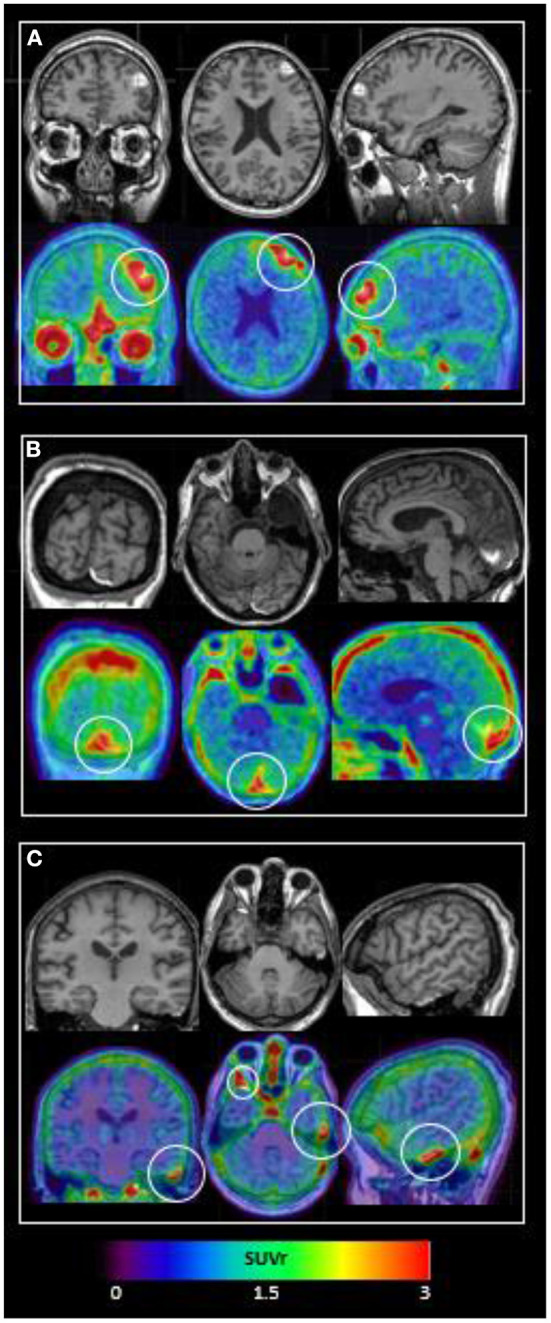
^18^F-MK6240 PET and MRI performed within 14 days of complicated mild TBI in 3 subjects. Top panel shows T1 MRI and bottom panel shows ^18^F-MK6240 PET (SUVr) overlaid on MRI. (A) Subject #1: MRI shows hemorrhagic contusions in L frontal and L>R orbitofrontal regions. PET shows focal radiotracer accumulation within and surrounding these regions, with the L frontal lesion (white circle) showing a c-shaped configuration. (B) Subject #2: MRI shows parafalcine trace subdural hematoma, diffuse atrophy, and a developmental arachnoid cyst. PET signal in the region of subdural hematoma (white circle) may be present, but is difficult to differentiate from (normal, off-target) signal in adjacent dura. (C) Subject #3: MRI shows R anterior and L lateral temporal epidural hematomas. PET shows extra-axial radiotracer accumulation in these regions (L temporal = small white circle; R temporal = larger white circle).
